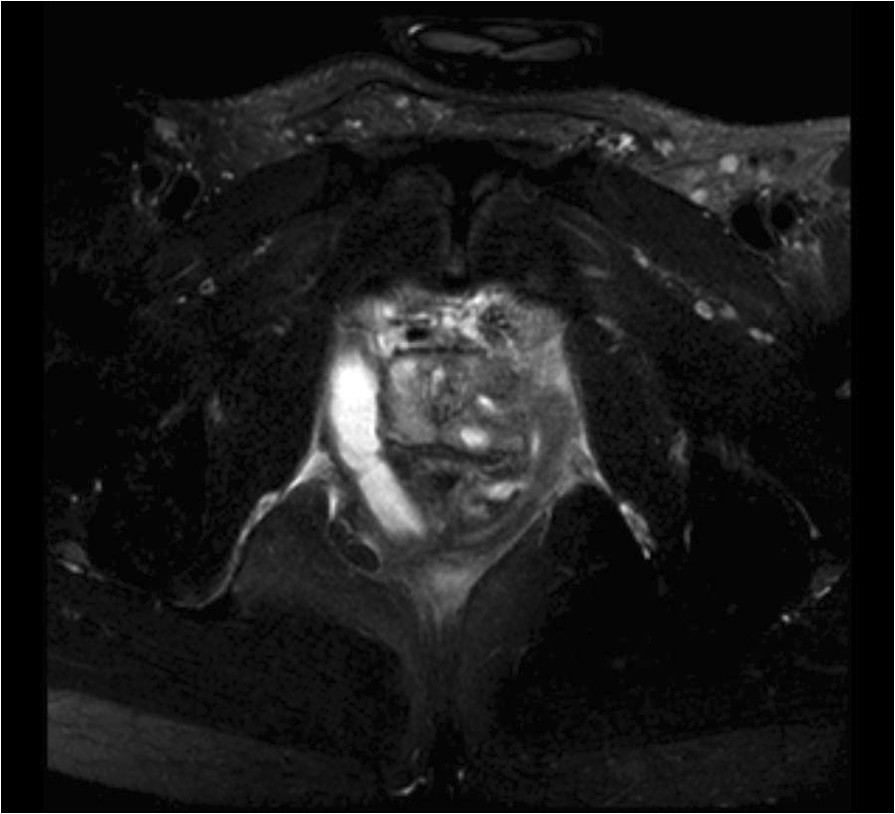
Pelvic MRI at D16. Control pelvic MRI at D16 showing regression of the abscesses in the left lobe and persistence in the right lobe.
